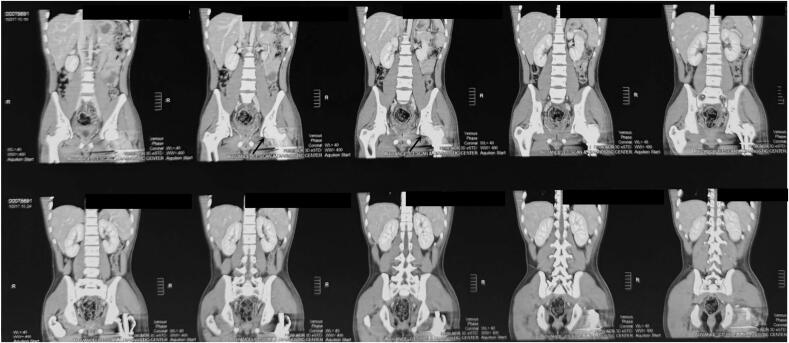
Post-operative CT scan of the pelvis and hip with linear, longitudinal and non-displaced fracture of head of left femur at the level of fovea capitis (Pipkin Type 1) marked by arrows.
